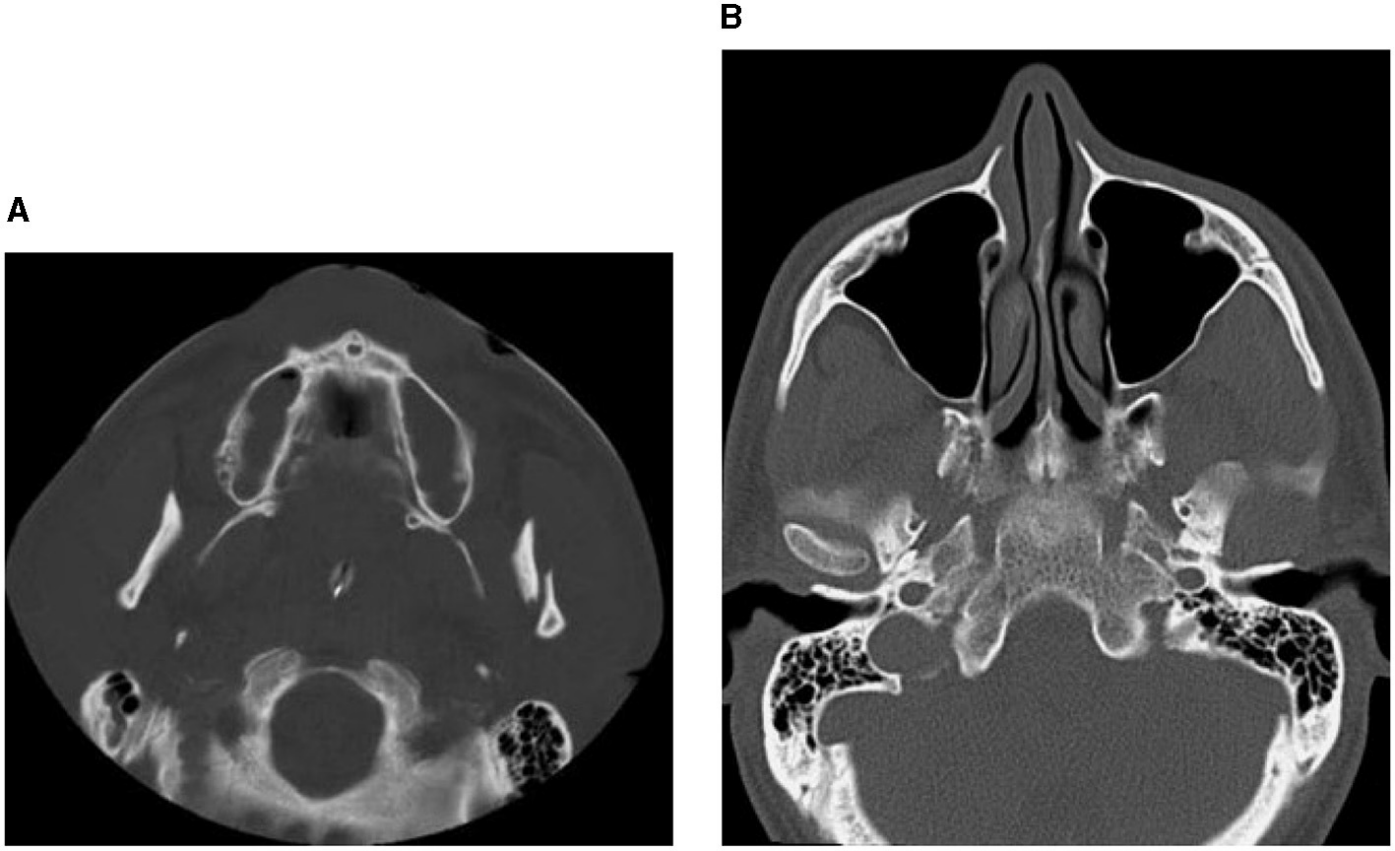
CT images showing factures of mandibular bones: a fracture of the left condyle, featuring lateral displacement of the proximal condylar fragment (A), and condylar fracture with dislocation (B).
